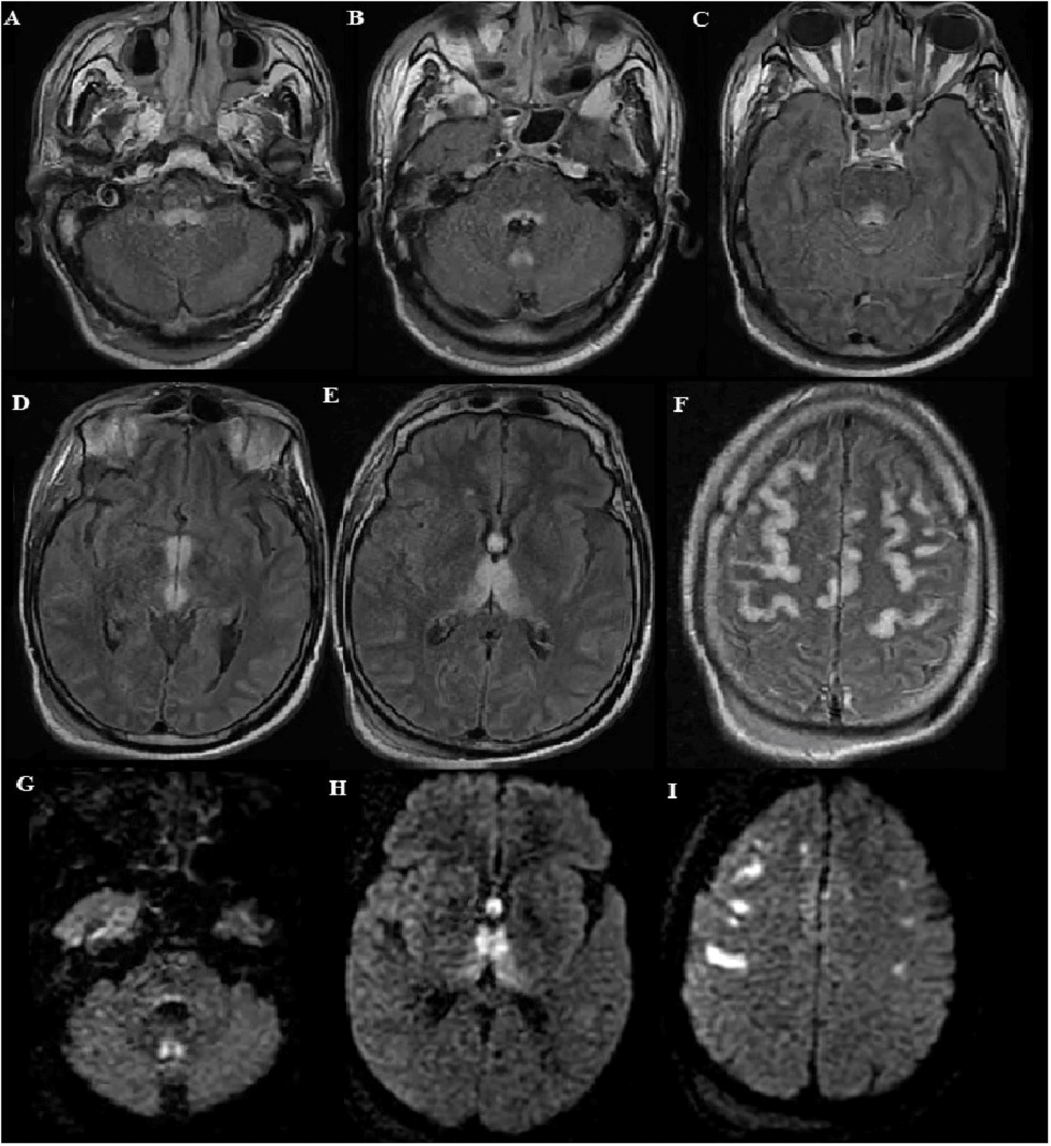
(A-I) FLAIR images (A-F) and diffusion weighted image (DWI) (G-I) of the brain: high signal lesions were seen in the bilateral mammillary bodies, tectal plate, periaqueductal gray matter of the midbrain, posterior pons, bilateral medial thalamic nuclei , midline cerebellum , and the cortical frontal ribbon FLAIR: fluid-attenuated inversion recovery
